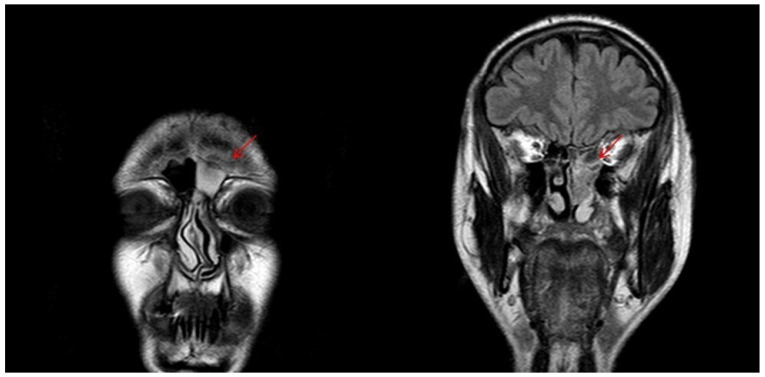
T2-weighted MRI image showing sinonasal neuroendocrine tumors—MENs of ethmoidal cells and to left frontal sinus before radiotherapy.
